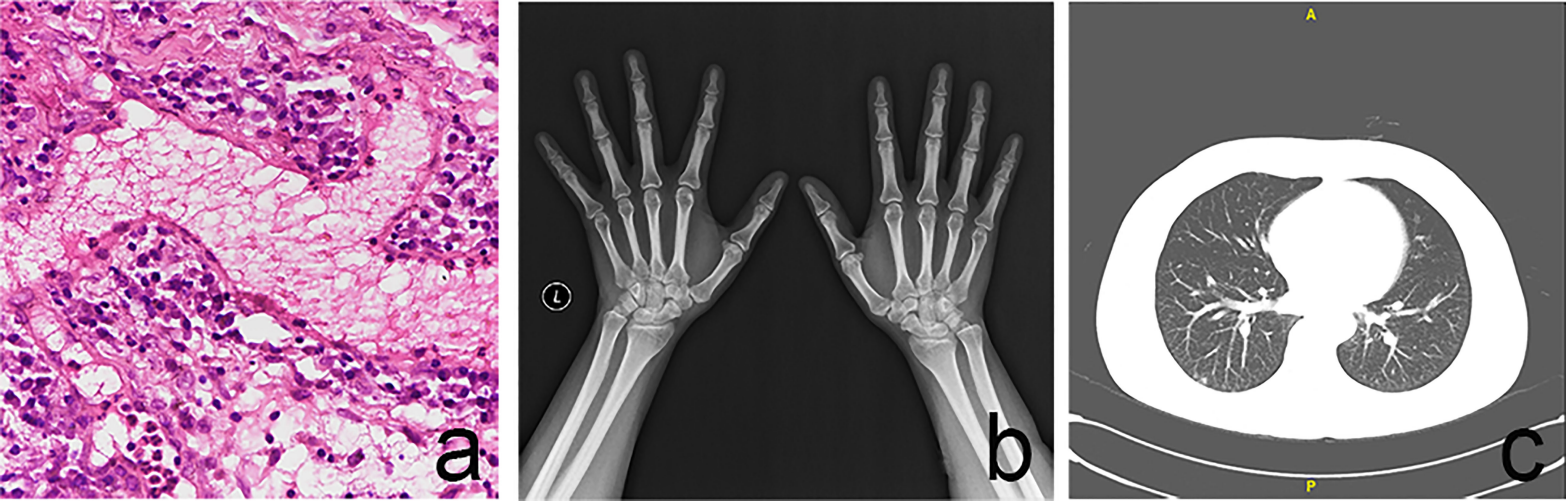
(A) The conjunctival biopsy of the right eye revealed vascular proliferation, telangiectasis, perivascular lymphocytes and plasma cells infiltration. (B) Hands and wrists X-rays with no evidence of bony erosion. (C) Chest CT showed multiple nodules in the inferior lobe of both lungs.
